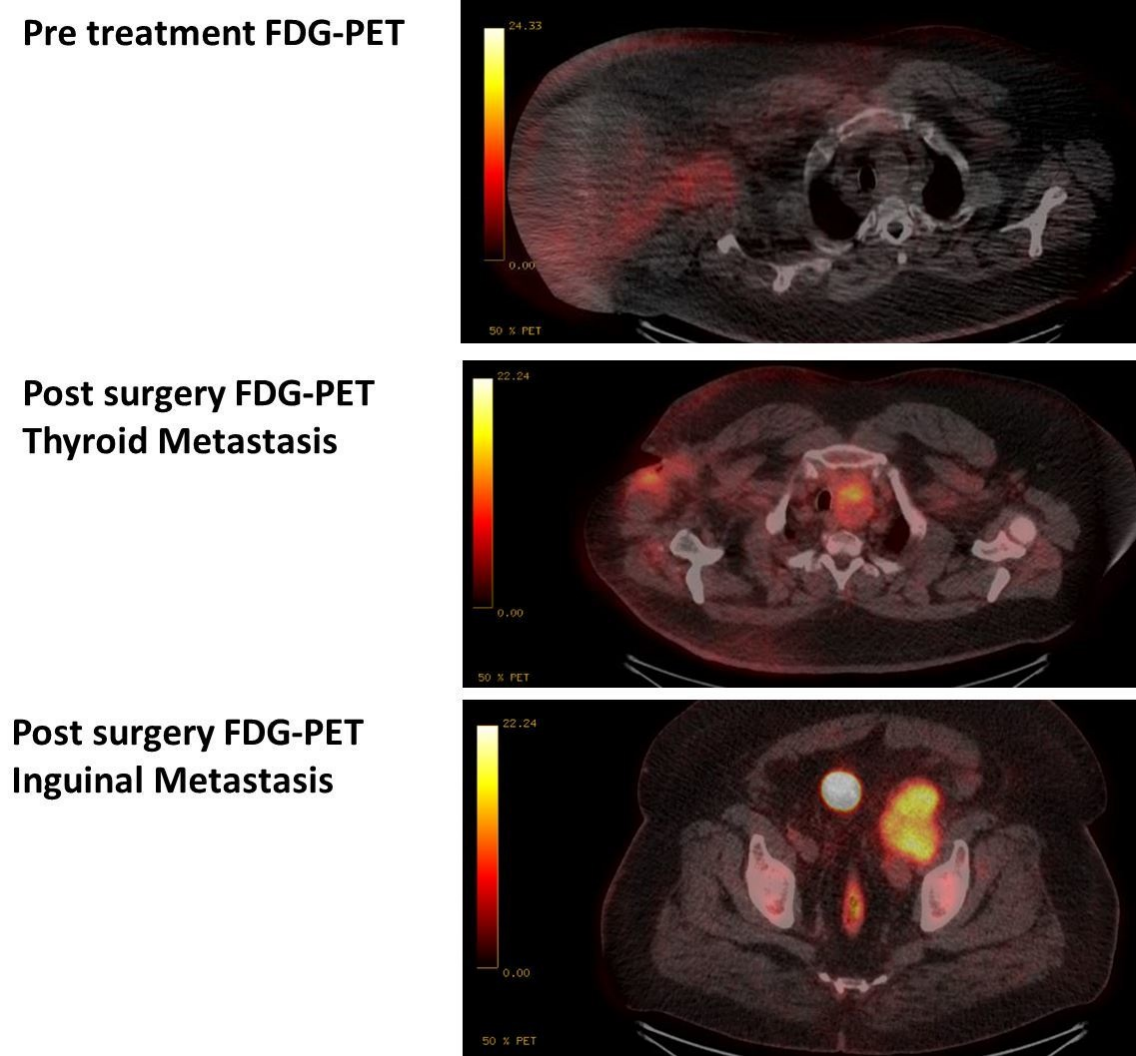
FDG-PET uptake prior to treatment and at the time of diagnosis of distant metastatic disease
